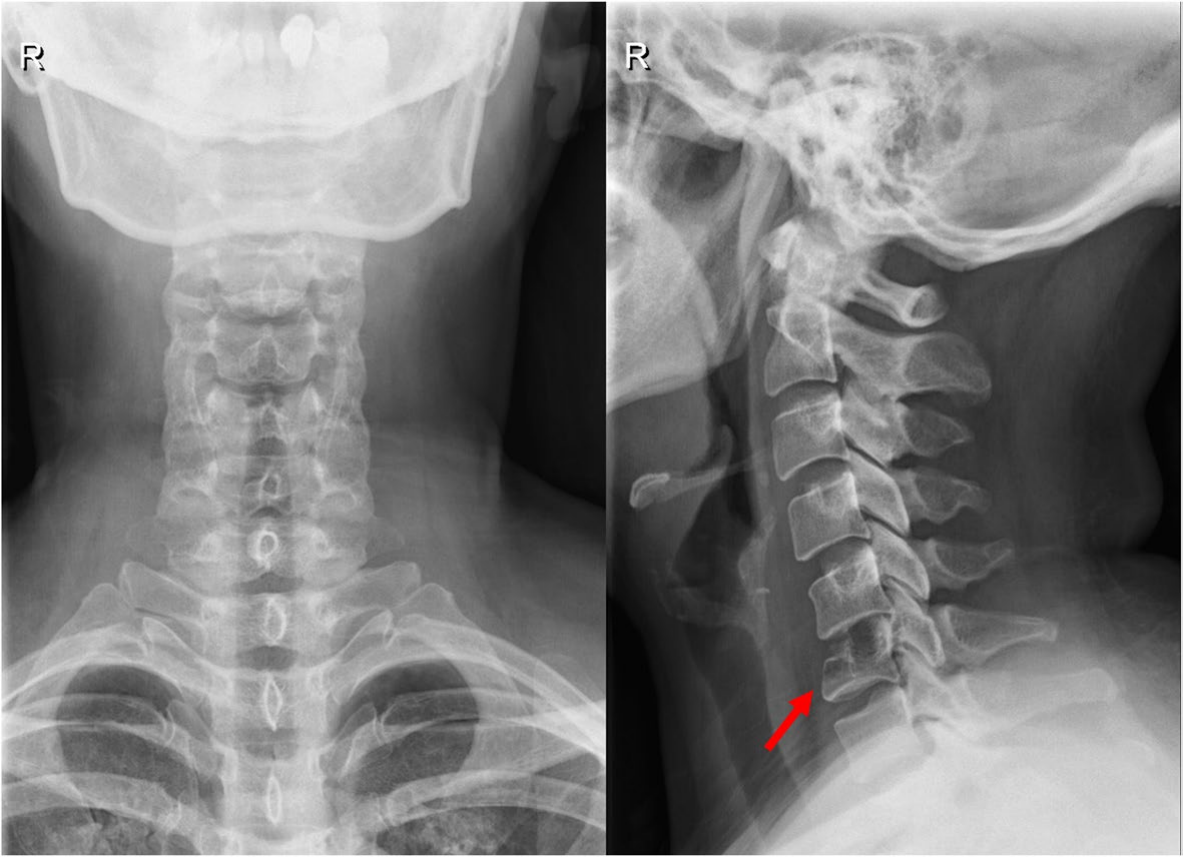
Cervical spine radiograph. A anterior–posterior view; B lateral view; arrow: C6 vertebral body
